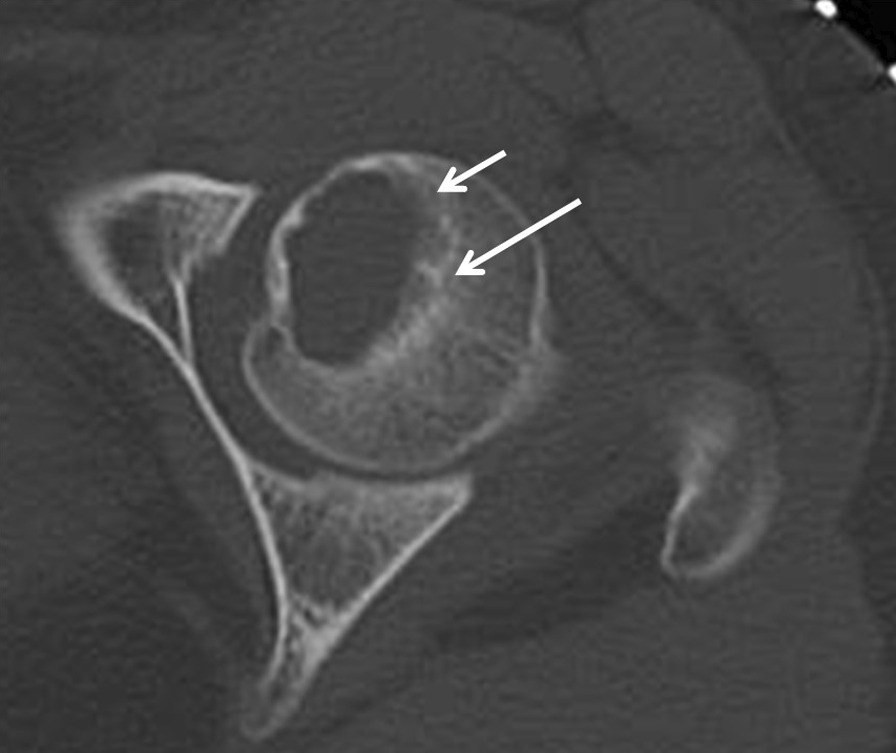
Preoperative computed tomography (CT) imaging: Case 1. CT images revealing a lytic lesion with a sclerotic rim located in the anteromedial side of the femoral head. The white arrow indicates the direction in which the osteotome was driven
